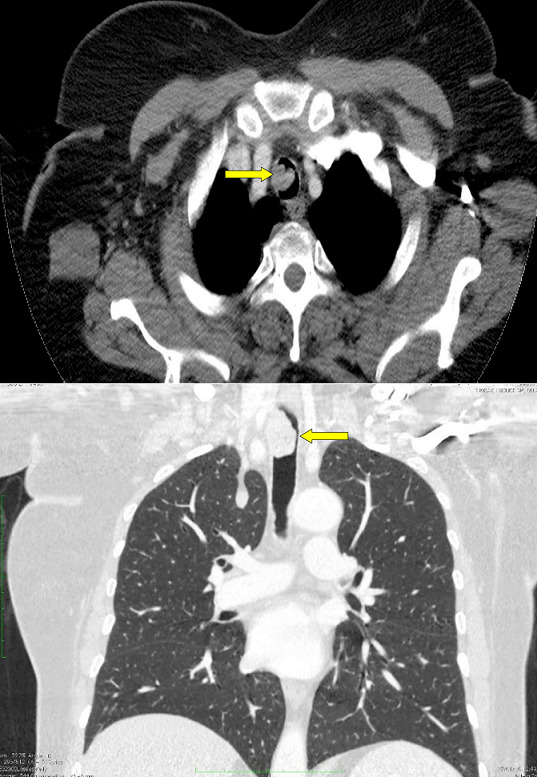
Chest CT scan - case 2: (A) axial CT scan image; (B) coronal CT scan image. Proximal tracheal solid mass (yellow arrow)
